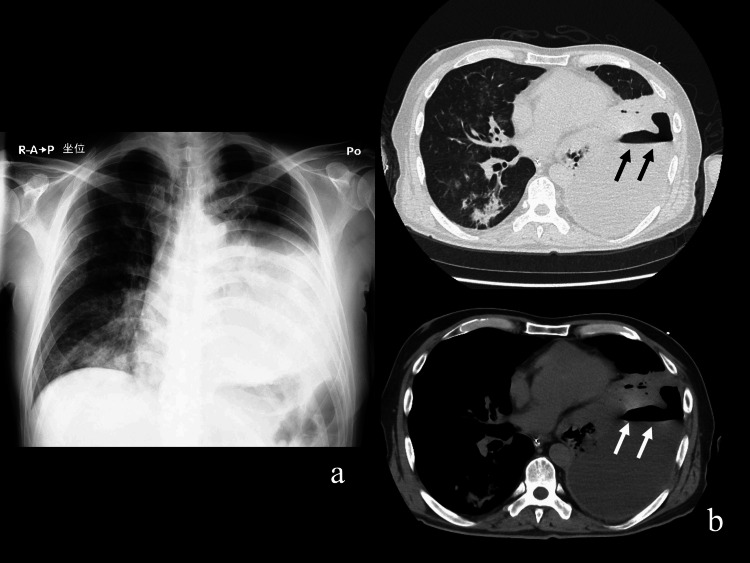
Imaging studies on admission. (a) Chest X-ray showing decreased radiolucency in the left thoracic cavity, suggestive of pleural effusion. (b) Chest computed tomography (CT) revealing a large left-sided pleural effusion with gas formation (arrow), consistent with empyema.
